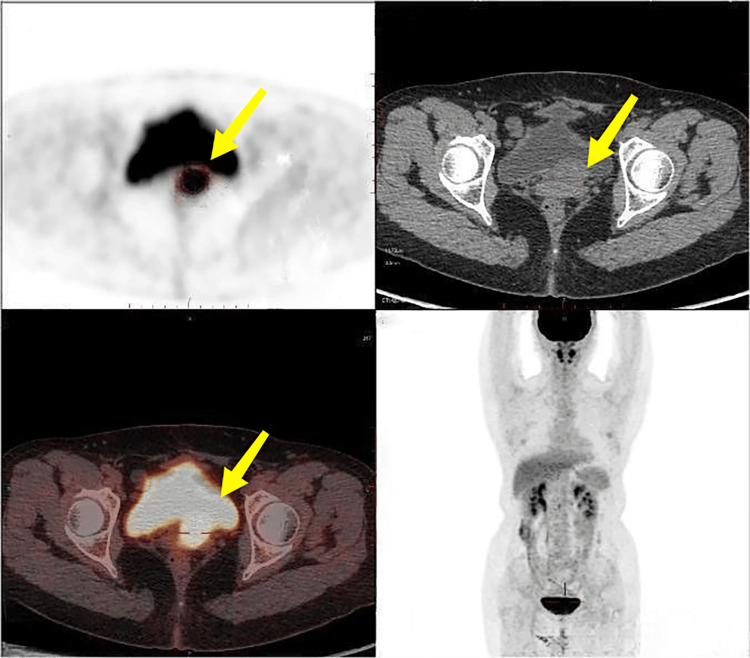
Positron emission tomography/computed tomography (PET/CT) findings Positron emission tomography/computed tomography showed a soft tissue density mass at the vaginal stump measuring approximately 3.3 cm × 3.1 cm with increased metabolism (yellow arrow).
